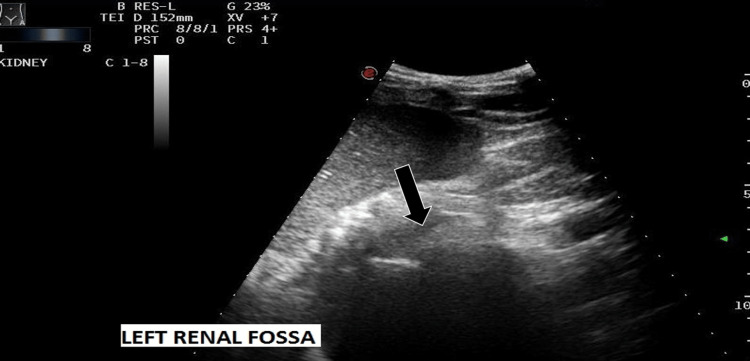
Abdominal ultrasound showing an absent left kidney in the left renal fossa (arrow)
